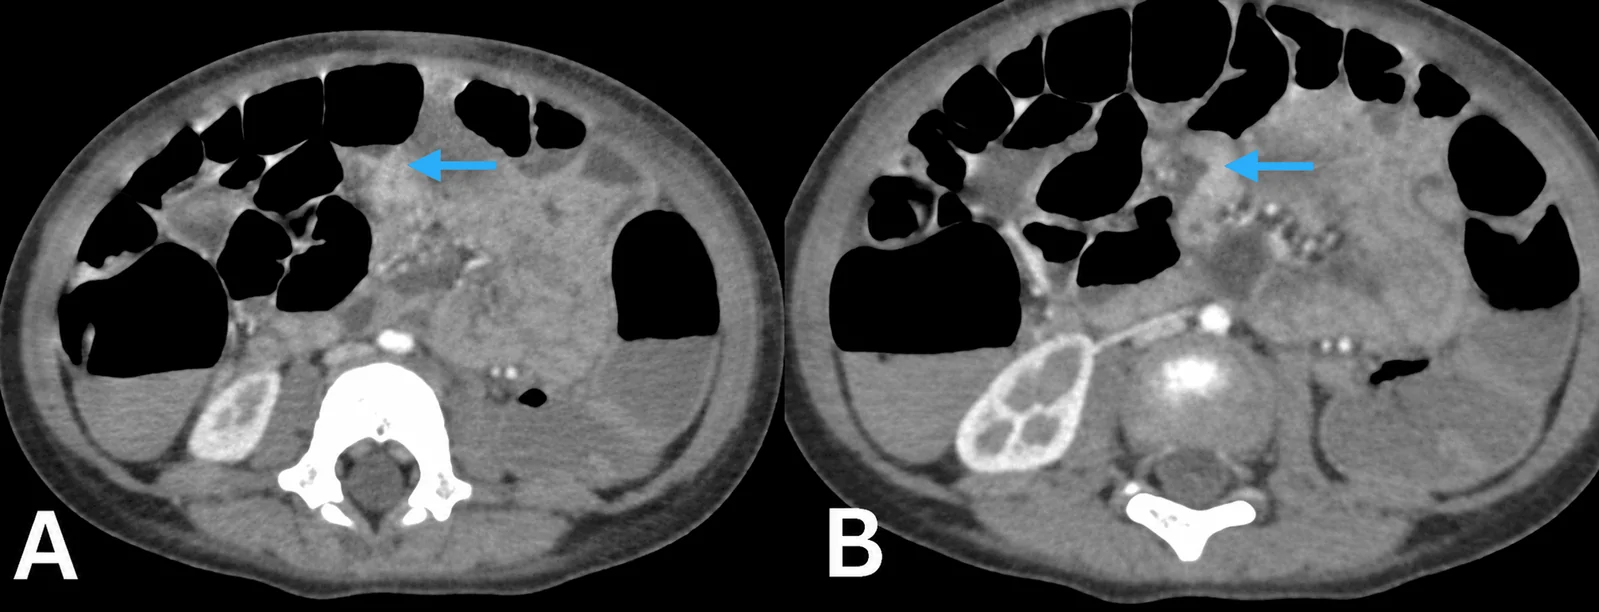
Contrast-enhanced computed tomography (CT) enterography demonstrating a blind-ending tubular structure arising from the mid-ileum (blue arrow in A and B). Axial image in (A) showing a suspicious tubular structure, further well-defined in (B), showing a well-demarcated tubular diverticular outpouching (blue arrows) arising from the small bowel.
